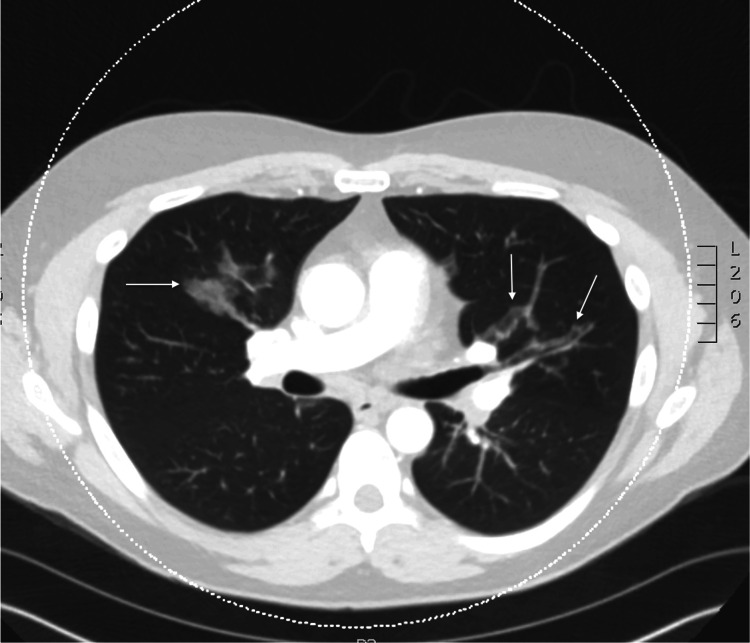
Axial view of non-contrasted chest CT demonstrating multifocal, predominantly peribronchial ground glass opacities (arrows).
